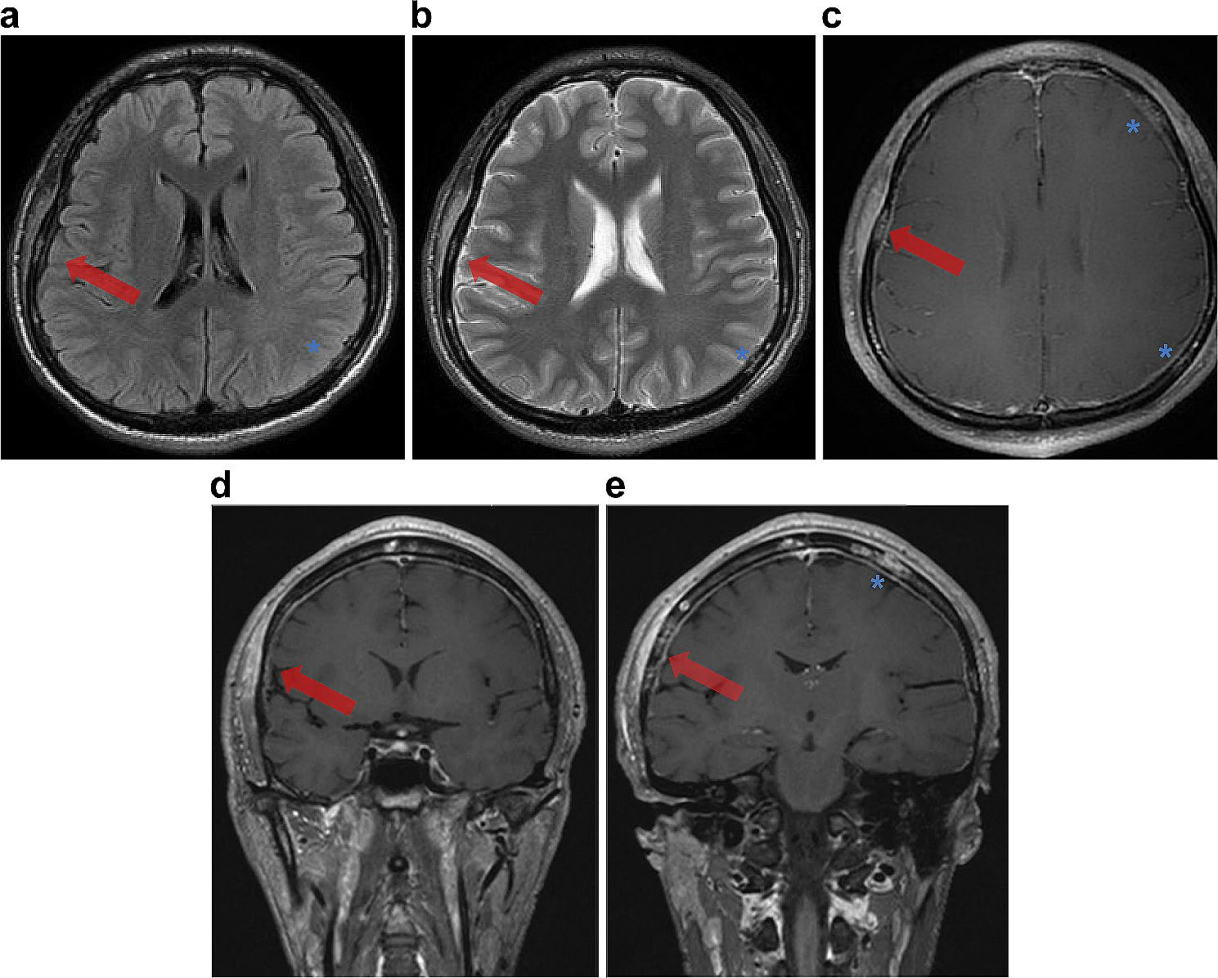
Hyperintense lesion (arrow) on T2WI (a) and FLAIR (b) in the right temporal bone with contrast enhancement on postcontrast T1WI (c, d, e), which is indicative of osteomyelitis. Adjacent soft tissues (including the temporalis muscle) were involved with swelling and hyperintensity on T2WI (a) and FLAIR (b) and enhancement on postcontrast T1WI (c, d, e). There were several hyperintense lesions on T2WI and FLAIR, and also contrast-enhanced lesions in the skull base. Dural enhancement was also observed (c, d, e), which is suggestive of meningitis
